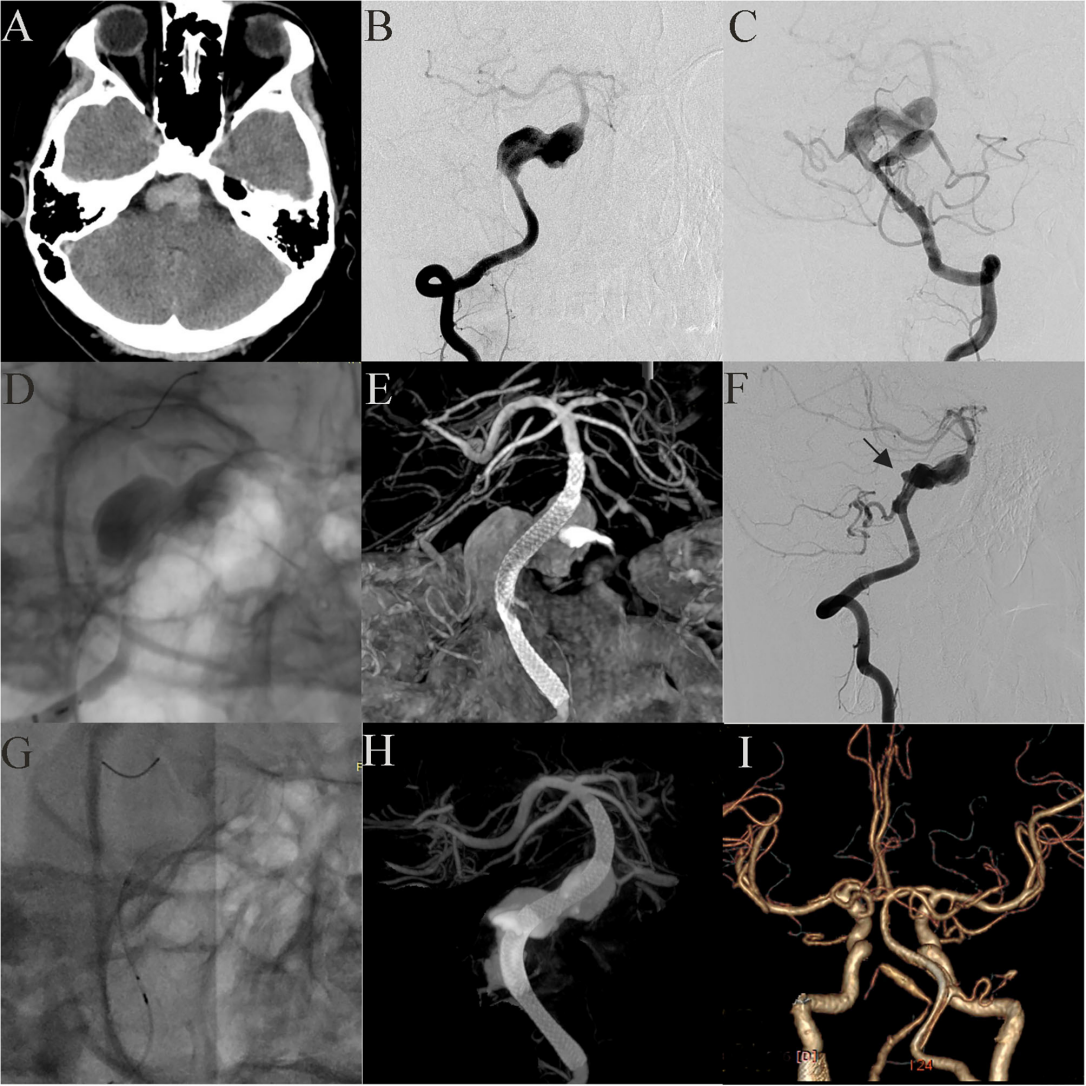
Case 21: A 17-year-old boy had a giant vertebrobasilar artery aneurysm and a PICA aneurysm (A, CT scanning; B, C, anteroposterior projections of bilateral vertebral arteries). A 3.75 mm × 35 mm PED was successfully deployed from the basilar artery to the right vertebral artery. Post-embolization angiograms showed significant contrast stasis (D) and good vascular reconstruction (E). The left PICA aneurysm was untreated. Two weeks later, the distal end of the stent (black arrow) retracted and fell into the aneurysm cavity (F). Another PED was used to bridge the original PED (G, H). Three months later, CTA examination showed that the aneurysm was completely occluded (I).
